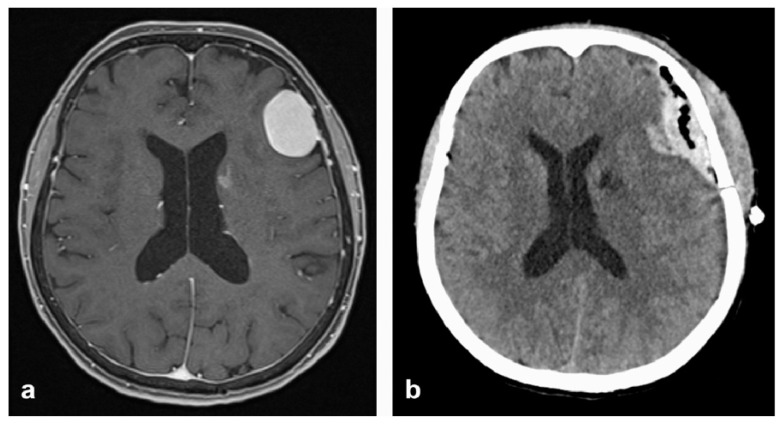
MRI (a) and CT (b) axial scans of head. Example of postoperative hemorrhage in the cavity after resection of a convexity meningioma.
